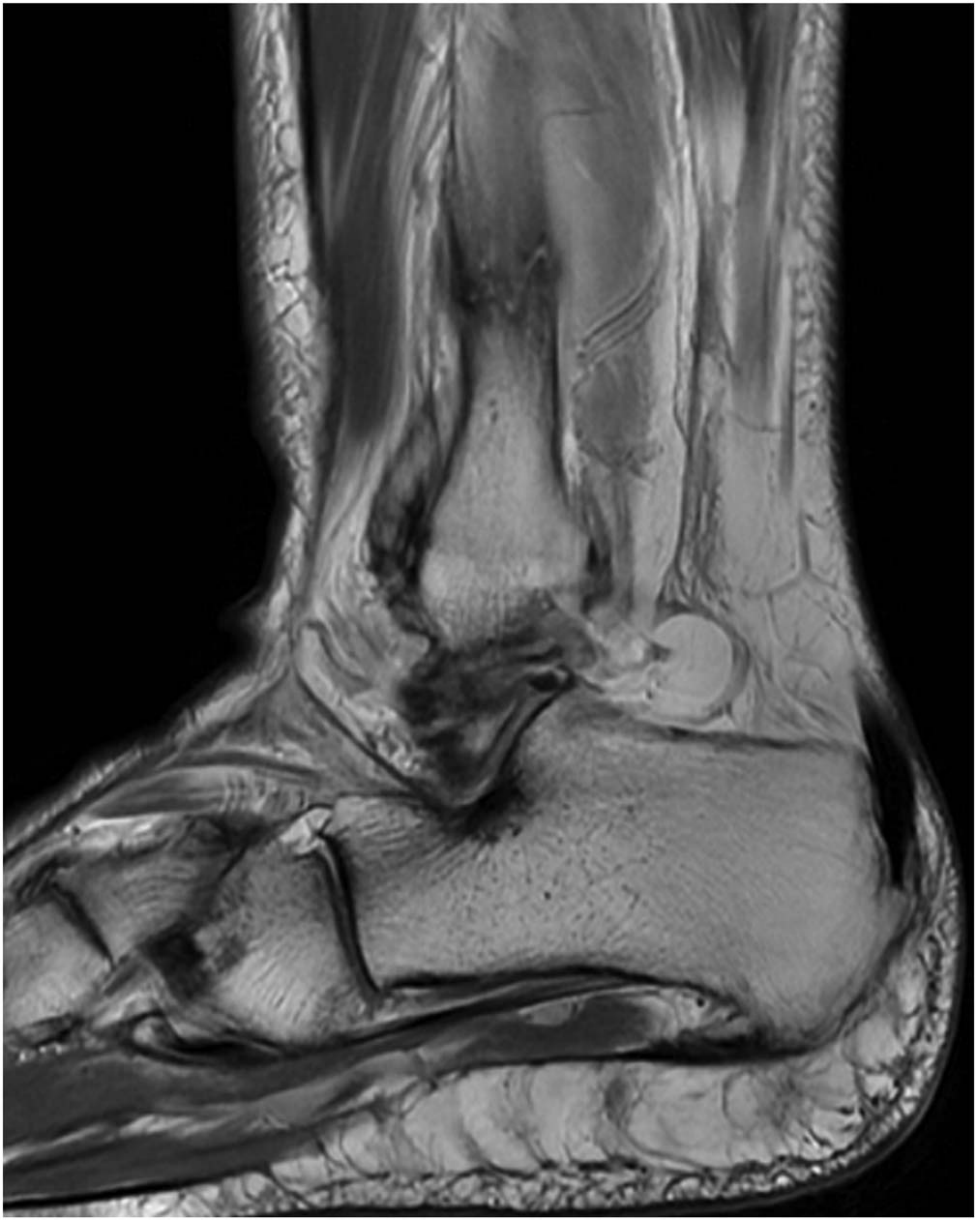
MRI demonstrating acute Weber C fibular fracture with partial-thickness tearing of the syndesmotic membrane of the right lower extremity.
